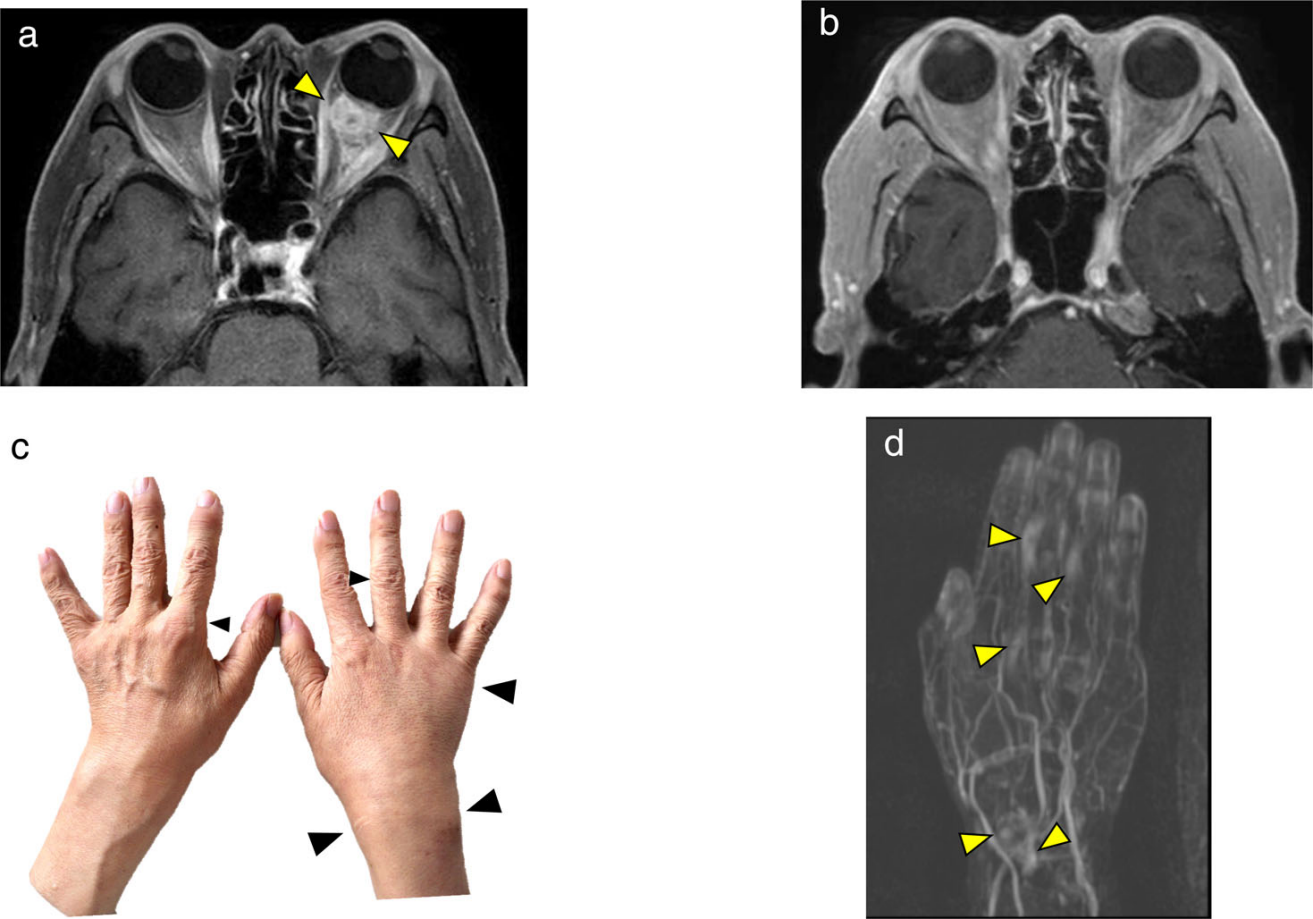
(a, b) The metastatic site in the left choroid had completely disappeared (arrows). (c) After 20 cycles of nivolumab, multiple joint pains and swelling occurred (left wrist and fingers, arrows). (d) Magnetic resonance imaging (MRI) revealed high signal intensity signs in the left wrist and fingers on dynamic gadolinium‐enhanced MRI (arrows).
